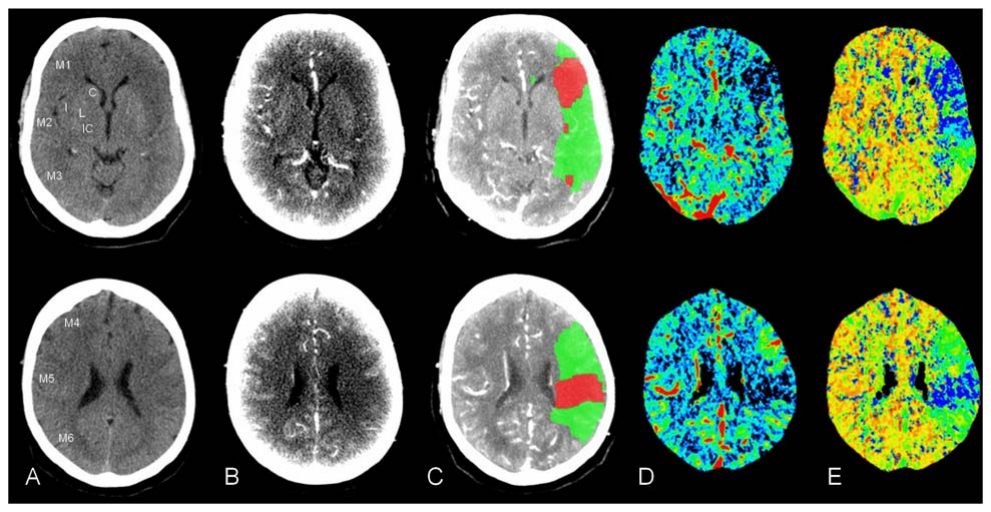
NCCT, CTA-SI and CTP in a patient with acute stroke. A 67-year-old male with aphasia and right-sided hemiparesis. Imaging approximately 1 hour after symptom onset. The upper row corresponds to the ganglionic ASPECTS level (C, caudate nucleus; L, lentiform nucleus; IC, internal capsule; I, insular region; M1–M3, cortical regions), the lower row to the supraganglionic ASPECTS level (M4–M6, cortical regions). Ischemic signs in the left MCA territory are seen on (A) NCCT; (B) CTA-SI; and on CTP in (C) penumbra and infarct maps; (D) CBV maps; and (E) MTT maps.
